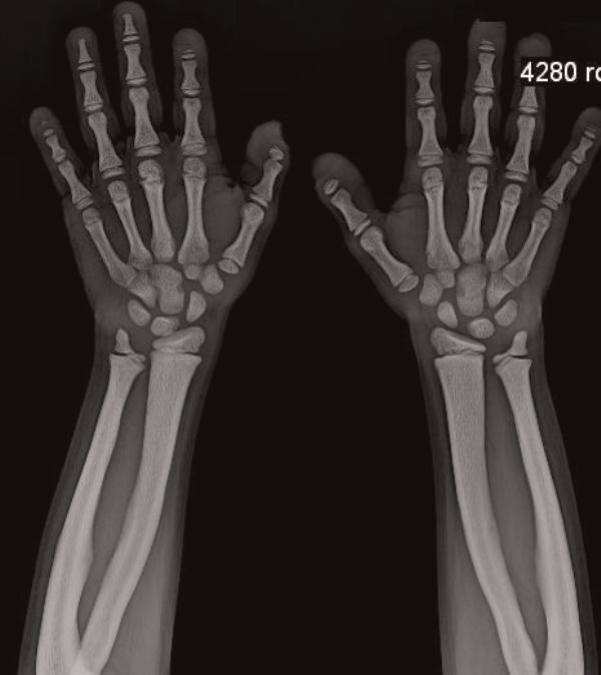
X-ray showing acroosteolysis hyperostosis with sparing of medullary cavity and normal bone age.
